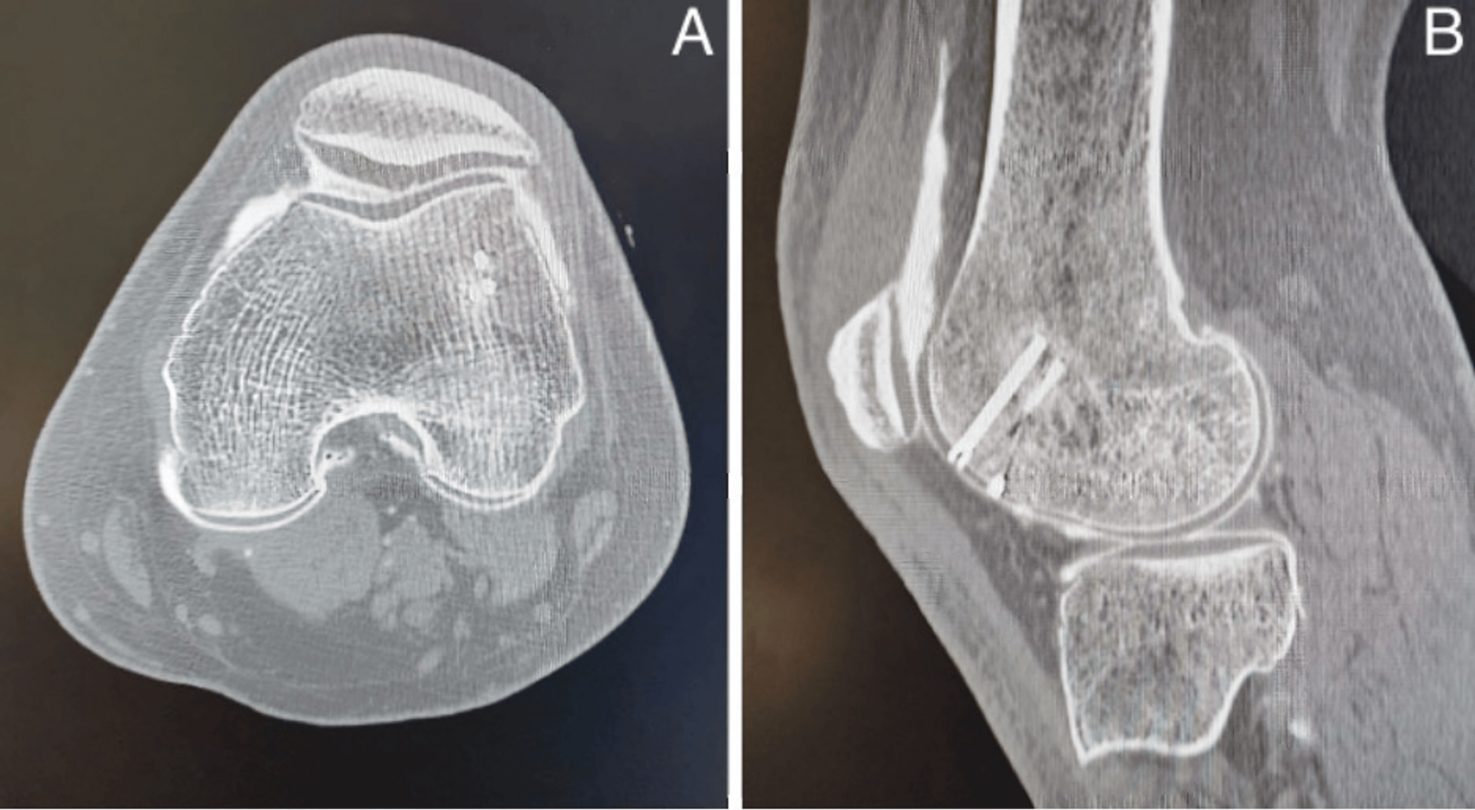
Follow-up arthro-CT scan at 1.5 years post-intervention showed consolidation of the osteochondral fragment, with no evidence of subsequent chondropathy or chondrolysis.
